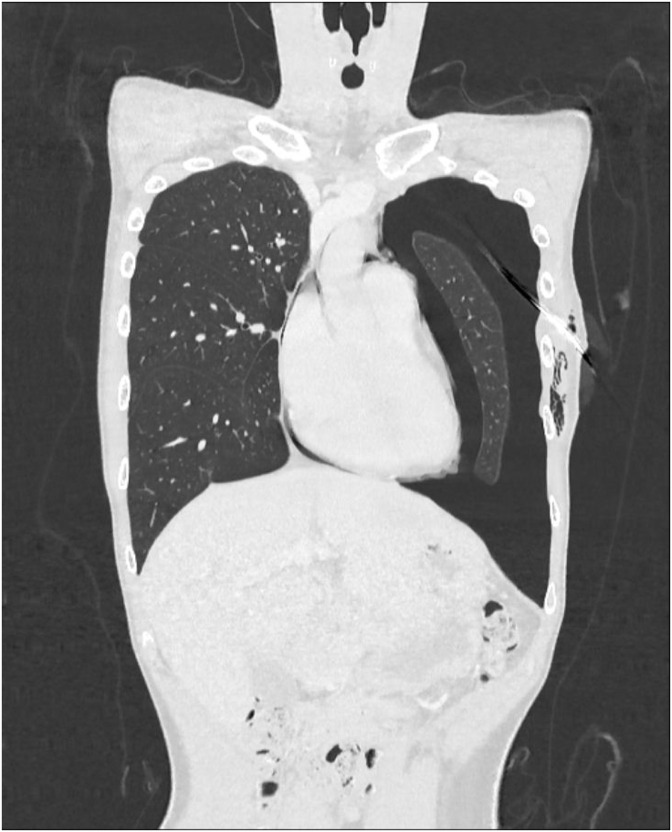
CT Thorax coronal view showing pneumomediastinum over the right heart border.
